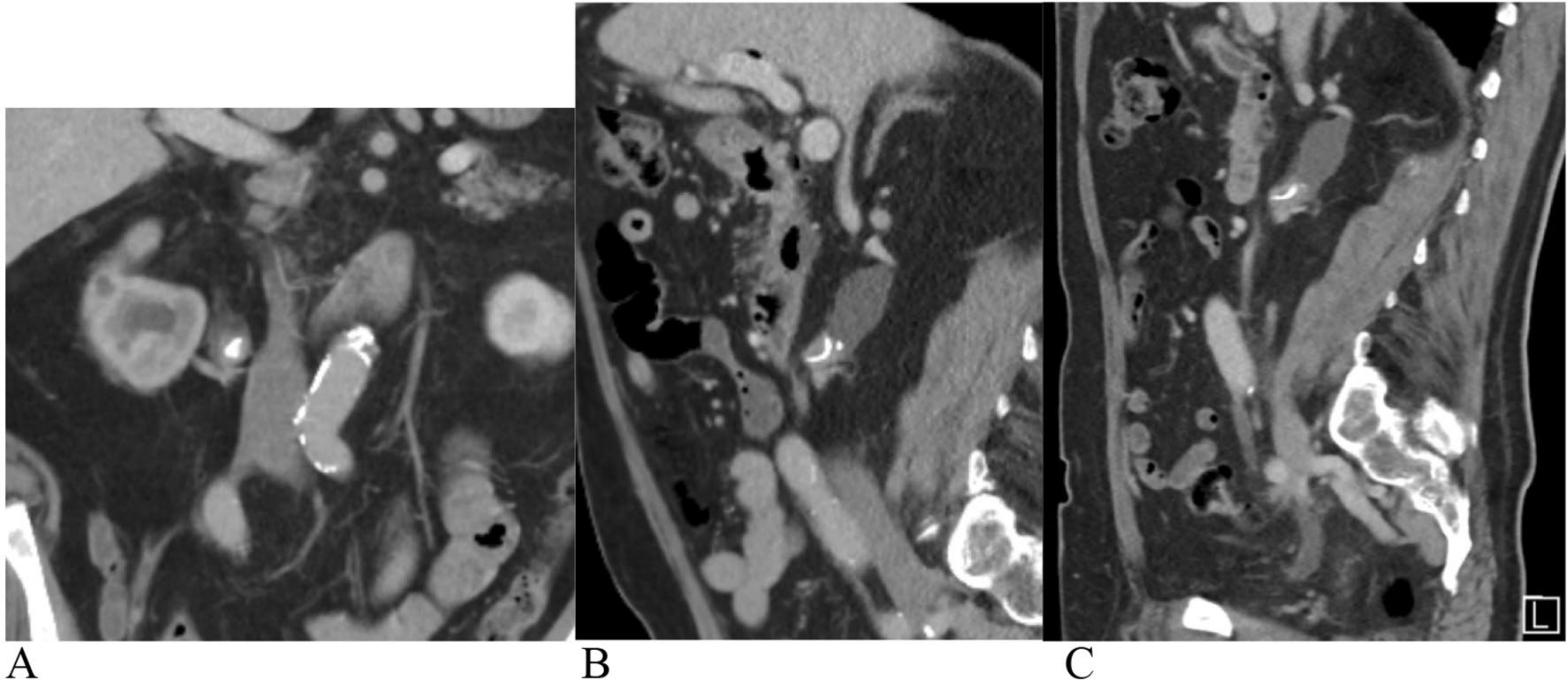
A 79-year-old man with right sided flank pain. No other symptoms. Previous smoker. A split bolus CT scan was performed. A calcification was found in the pelvoureteric junction (PUJ) and the patient was diagnosed as having a renal stone. Split bolus CT scan has a native phase and then contrast is given as two doses, so that there are simultaneous venous and excretion phases, which is not optimal to find urothelial tumours. After 2 months, the patient was referred to the urology department and a multiphase CTU was performed. a Split bolus coronar view. A calcification can be seen in the PUJ. b Split bolus sagittal view. A calcification can be seen; however, it is formed like an eggshell. c Multiphase CTU, sagittal view. A contrast loading tissue mass can be seen around the calcification. URS revealed a tumour with calcification on the surface
